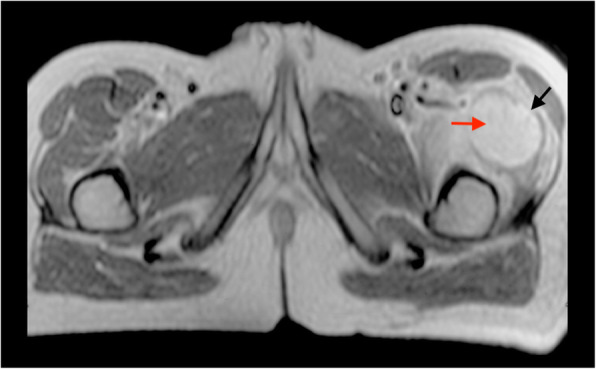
Contrast T1-weighted axial MRI: Well-defined contrast-enhanced lesion (5 × 4 × 3 cm) within the proximal third of the left vastus lateralis (red arrow) sparing the cortical bone and separated from surrounding oedema by a hypointense rim (black arrow)
